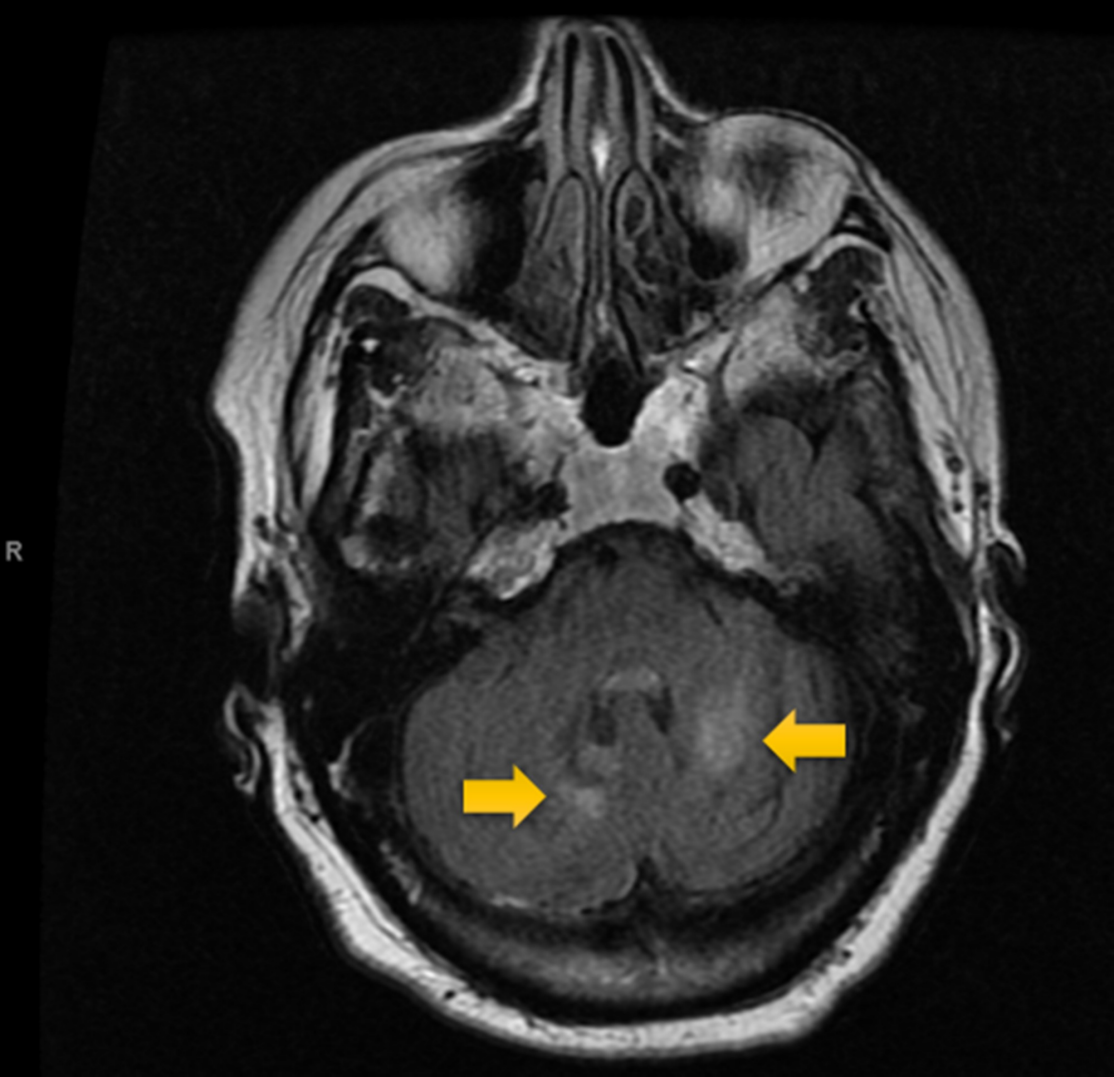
T2-flair MRI Scan of the Head Non-contrast T2-flair MRI showing old infarcts bilaterally in the inferior cerebellar hemispheres.
